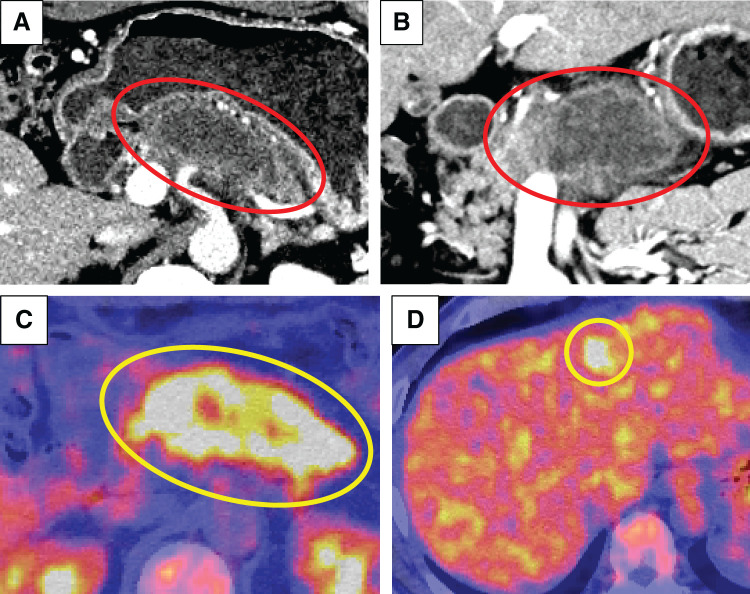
Contrast-enhanced CT and PET-CT were performed upon initial diagnosis. (A, B) The red circle shows the primary tumor. The yellow circle shows significantly abnormal uptake in (C) the primary tumor and in (D) the left lobe of the liver.
